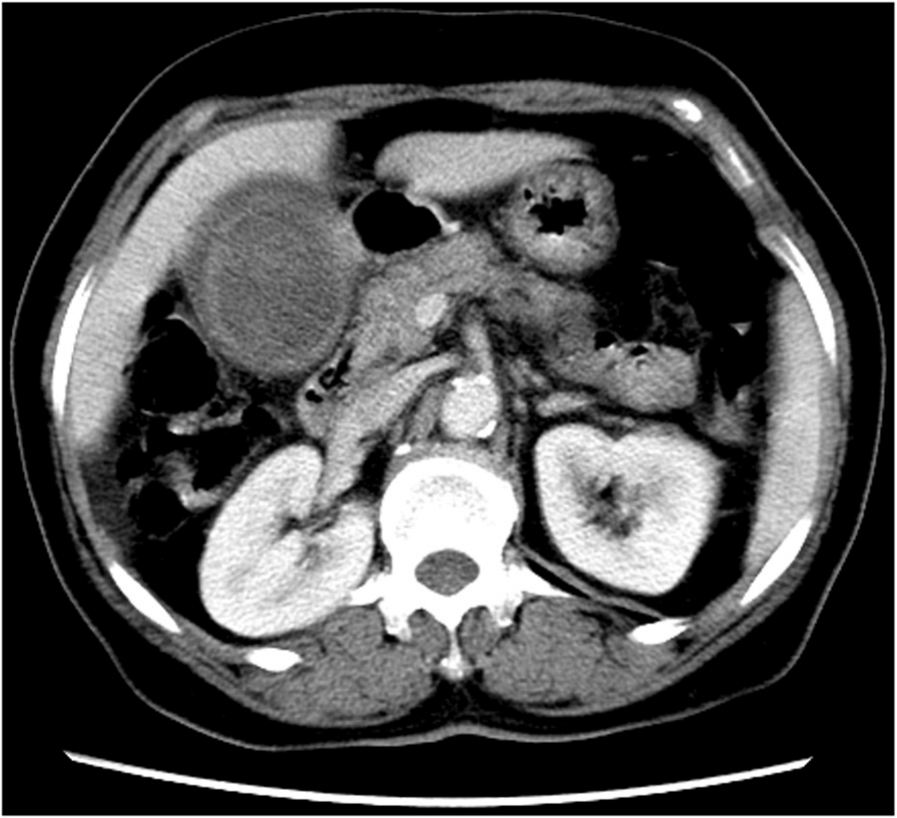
Enhancement computed tomography (CT) showed soft tissue tumor and manifest intensification.
